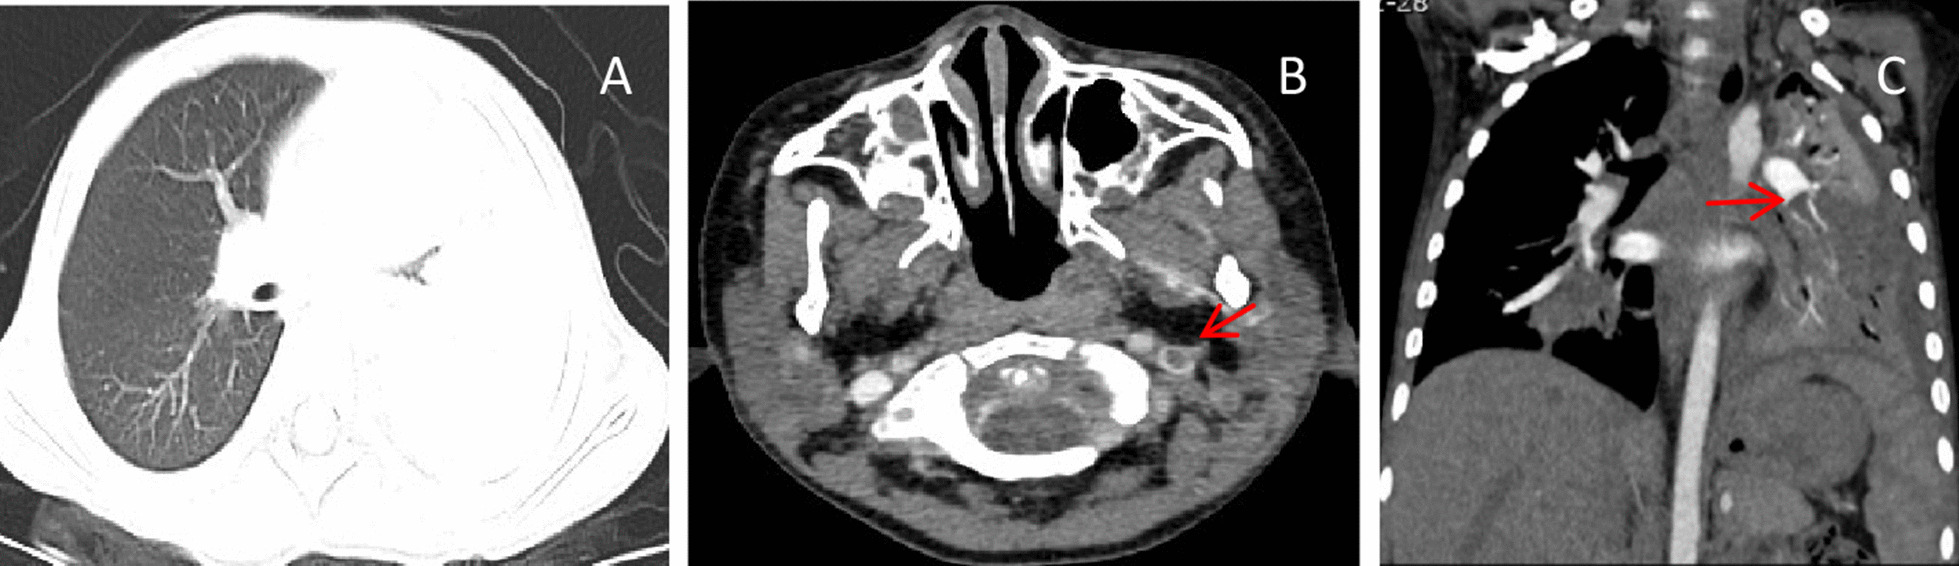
A Chest imaging revealed consolidation with high density in the left lung. B Neck CTA showed a filling defect in the left internal jugular vein. C Chest CTA showed a filling defect in the left pulmonary artery
